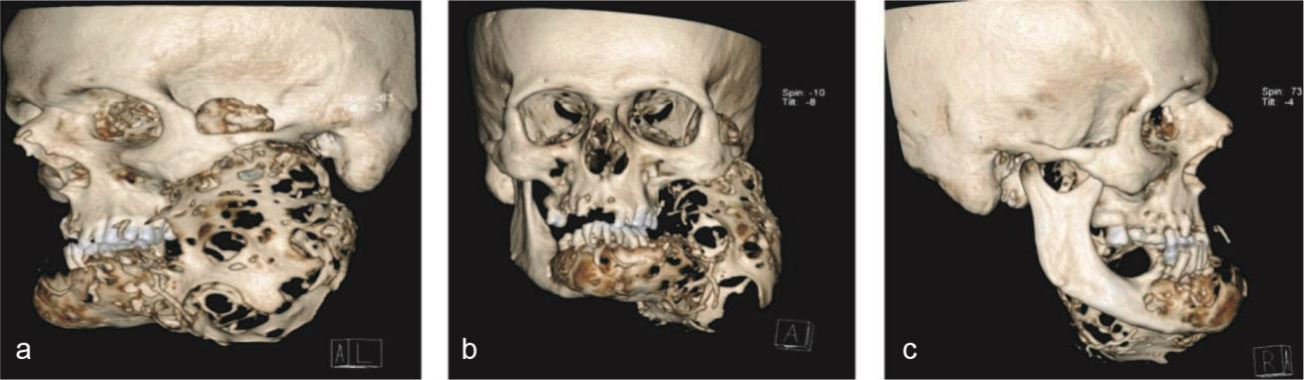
Preoperative computed tomography of the lesion (a: Frontal, b: Left, c: Right)
